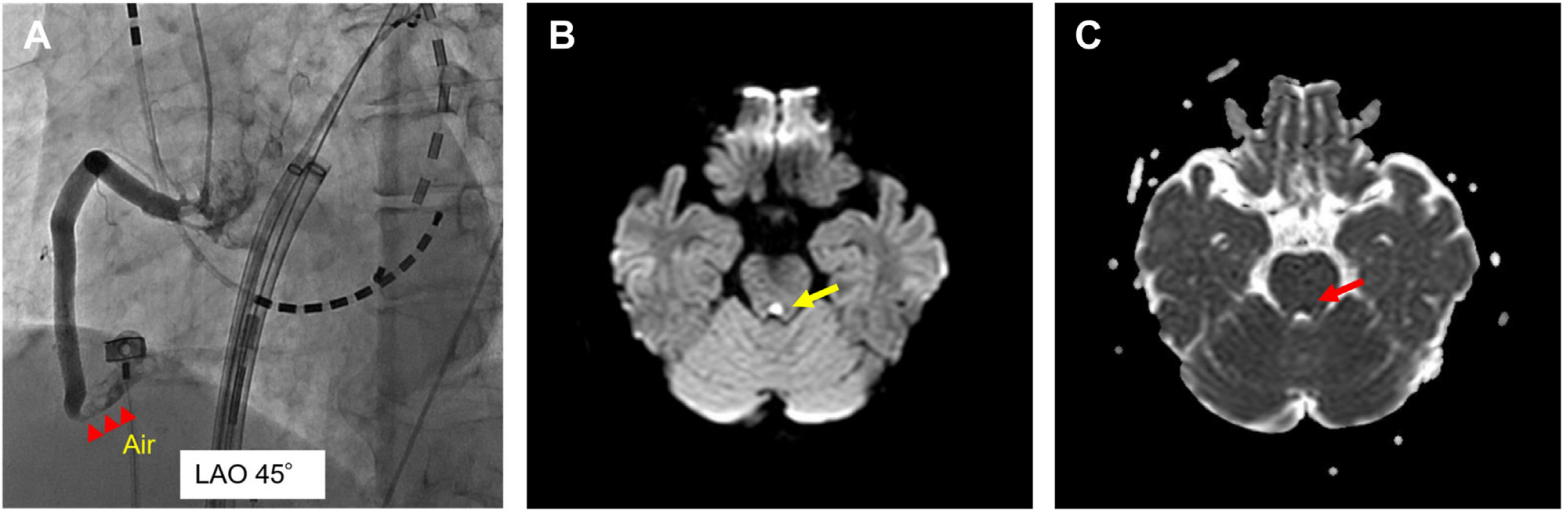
A: During the insertion of the balloon through the guiding sheath (Treswaltz; Toray Industries, Inc, Tokyo, Japan), angiography showed a right coronary artery occlusion due to massive air bubbles (*red arrowheads*). LAO = left anterior oblique. B: Diffusion-weighted magnetic resonance imaging of an acute stroke shows an infarct in the pontine tegmentum (*yellow arrow*). A low signal is seen on the apparent diffusion coefficient map (red arrow).
